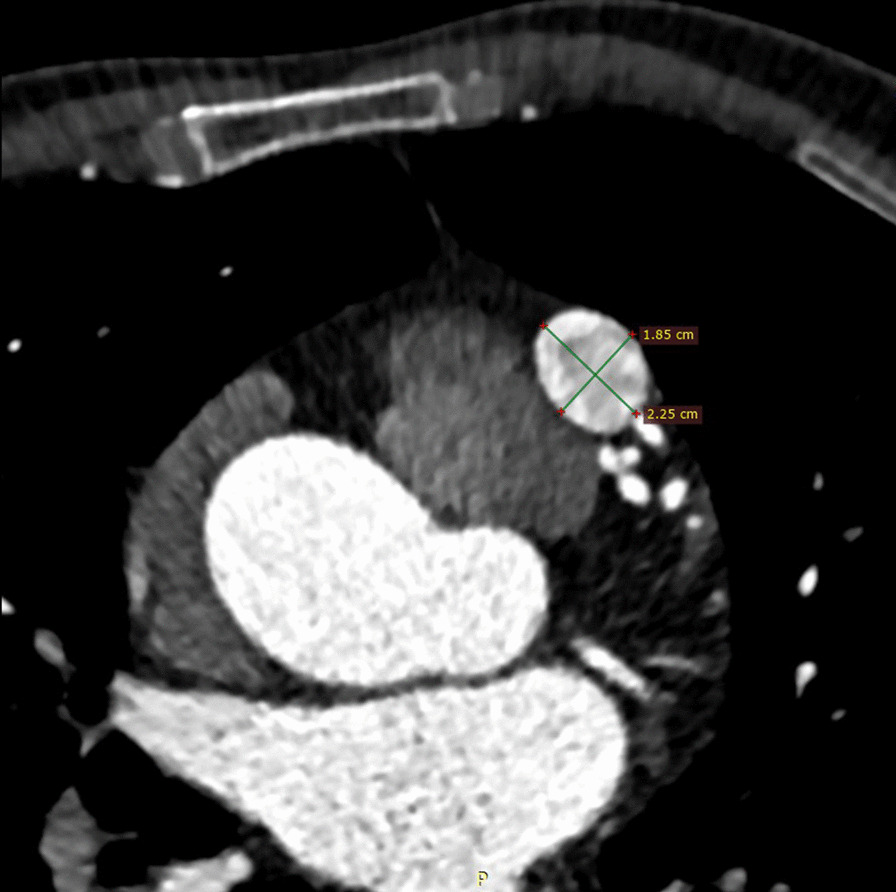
Coronary computed tomography angiogram in the local hospital spotted a coronary aneurysm of 2.23 * 1.81 cm
